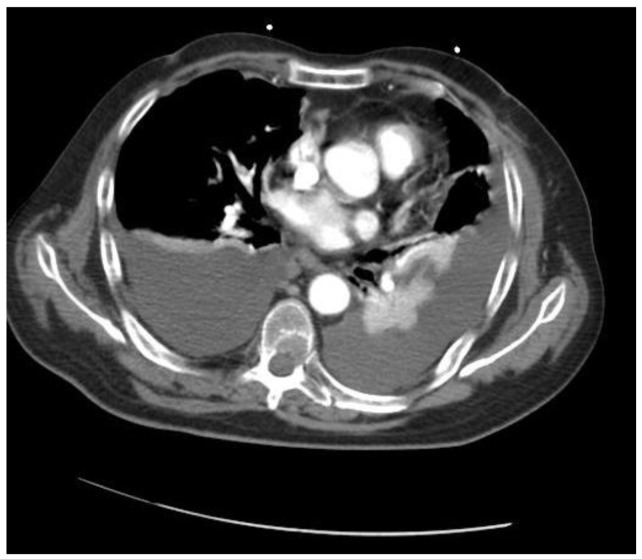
Angio CT-scan showing the metastatic lesions from GBM in the left inferior lobe and in the pleural cavity.
